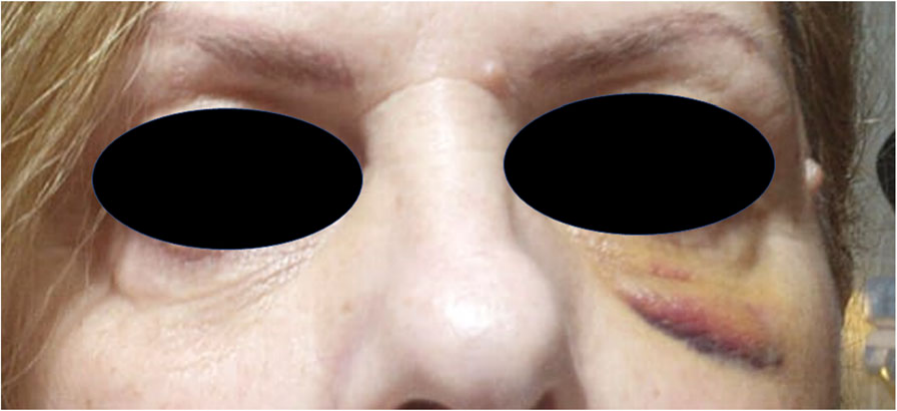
CT image after surgery showing the zygomatic implant tip in the lateral orbit
